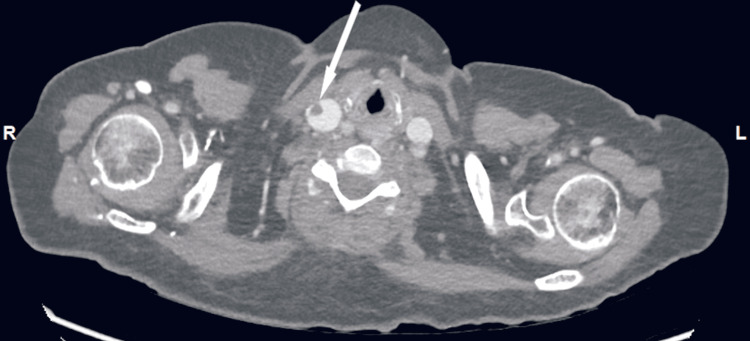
CT thorax, abdomen and pelvis, axial view (Case 3). Arrow shows thrombus extending into the right jugular vein.
